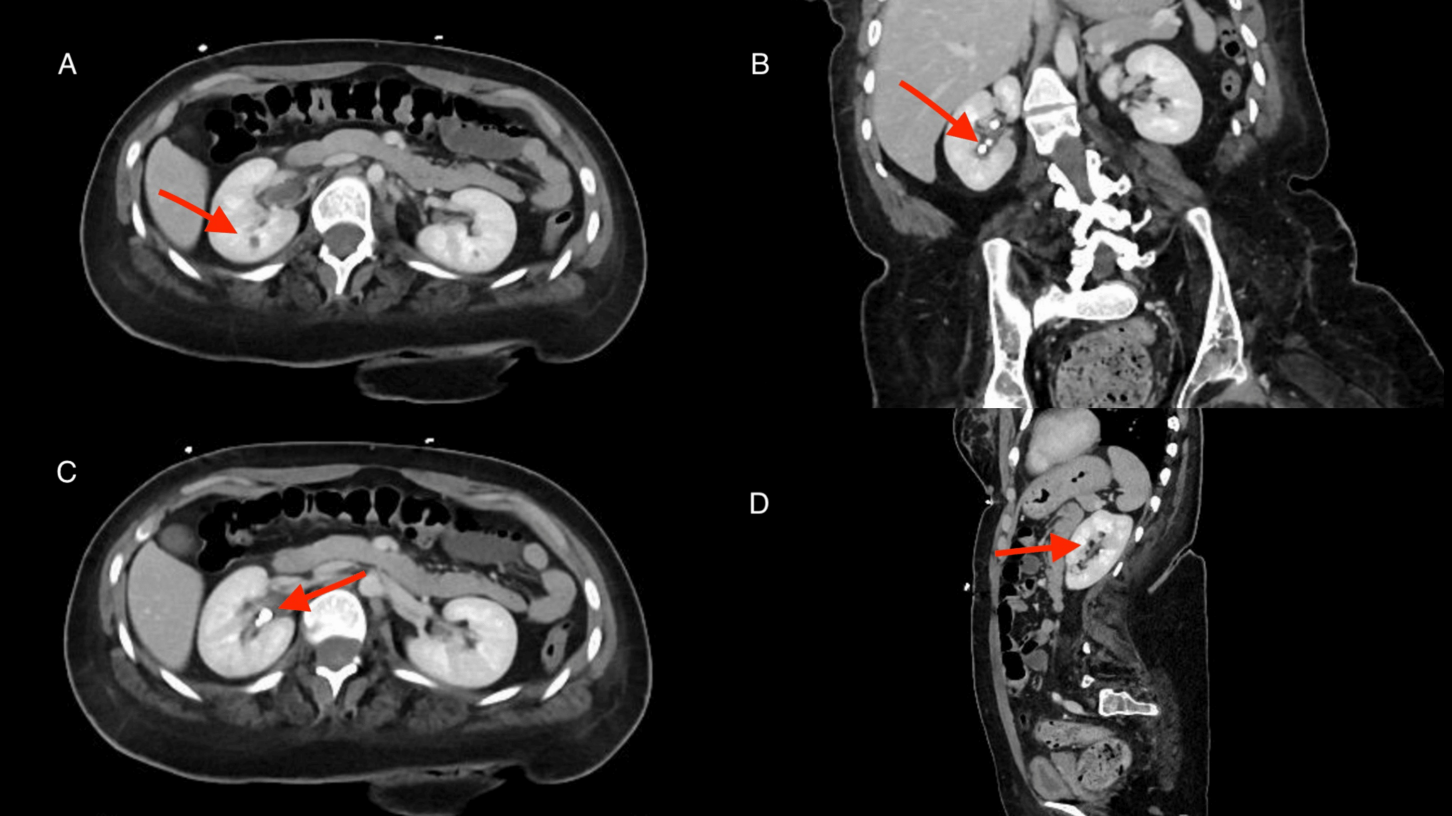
Contrast-enhanced CT abdomen and pelvis demonstrating acute pyelonephritis and right nephrolithiasis. (A) Axial contrast-enhanced CT with right perirenal fat stranding and a hypoenhancing cortical focus in the anterior mid-right kidney, consistent with pyelonephritis. (B) Coronal reconstruction showing multiple right renal calculi. (C) Axial CT with non-obstructive right renal stones, pyelonephritis without obstruction. (D) Sagittal reconstruction with longitudinal view of the right kidney with a visible region of focal hypoenhancement consistent with pyelonephritis.
